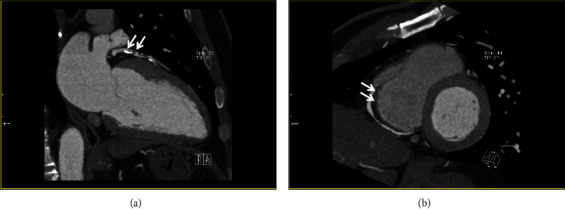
Coronary computed tomography angiography. (a) Left arterial descending artery proximal, mixed plaque with 70–80% stenosis and positive remodeling (white arrows). (b) Right coronary artery (RCA) proximal, mixed plaque with 50–70% stenosis and positive remodeling; RCA mid, mixed plaque with focal near total occlusion; and RCA distal, noncalcified plaque with 40% stenosis (white arrows).
